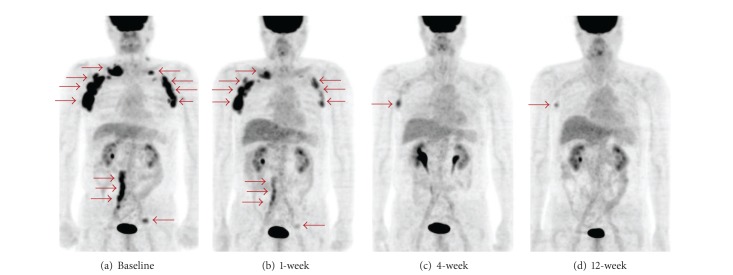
Maximum intensity projection images of patient 3. (a) Baseline, (b) 1-week scan, (c) 4-week scan, and (d) 12-week scan. FDG-avid lesions (arrow) are seen in bilateral subclavicular, axillary, abdominal para-aortic, and left inguinal area at baseline. Decrease in metabolism is observed at 1 week. All lesions continued to decrease to 12 weeks. At 12 weeks, only slight accumulation in the right axillary lesion is visible. Physiological activities are seen in the liver and the urinary tract.
